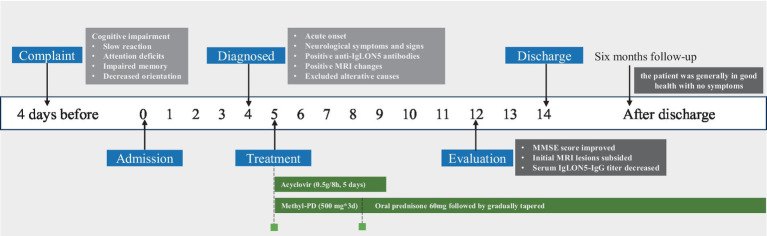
Timeline displaying the evolution of this case: in the white boxes, the date has been reported; in the blue boxes is a list of the important events that have been performed; in the green boxes, we have displayed the therapies; and in the black boxes, the evaluation was conducted, MRI, magnetic resonance imaging; Methyl-PD, methylprednisolone; MMSE, Mini-mental stare examination.
